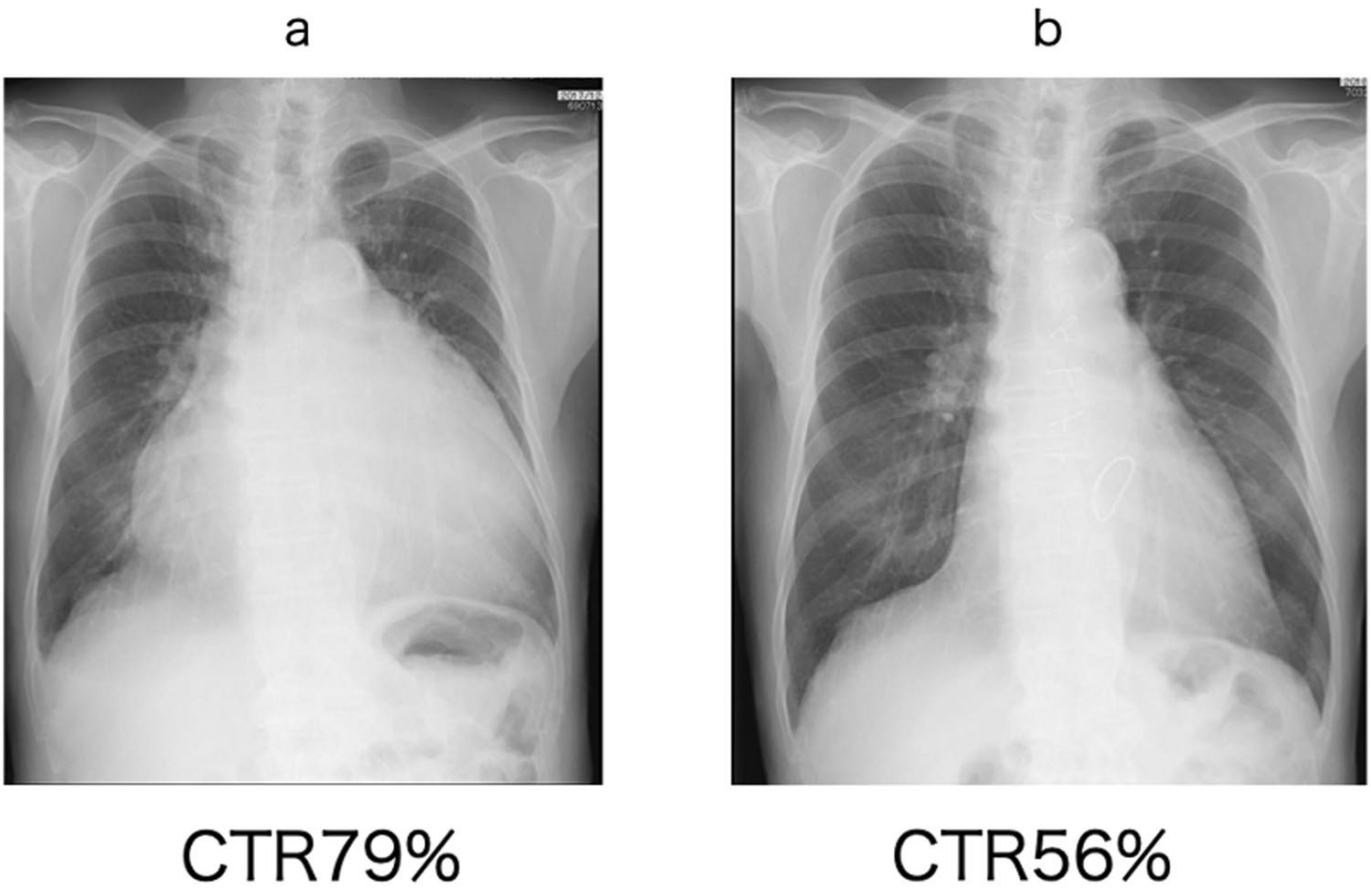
Chest radiographs (a) before surgery and (b) 3 months after an integrated operation involving mitral annuloplasty with patch augmentation, biatrial plication, and tricuspid annuloplasty. *CTR* cardiothoracic ratio
